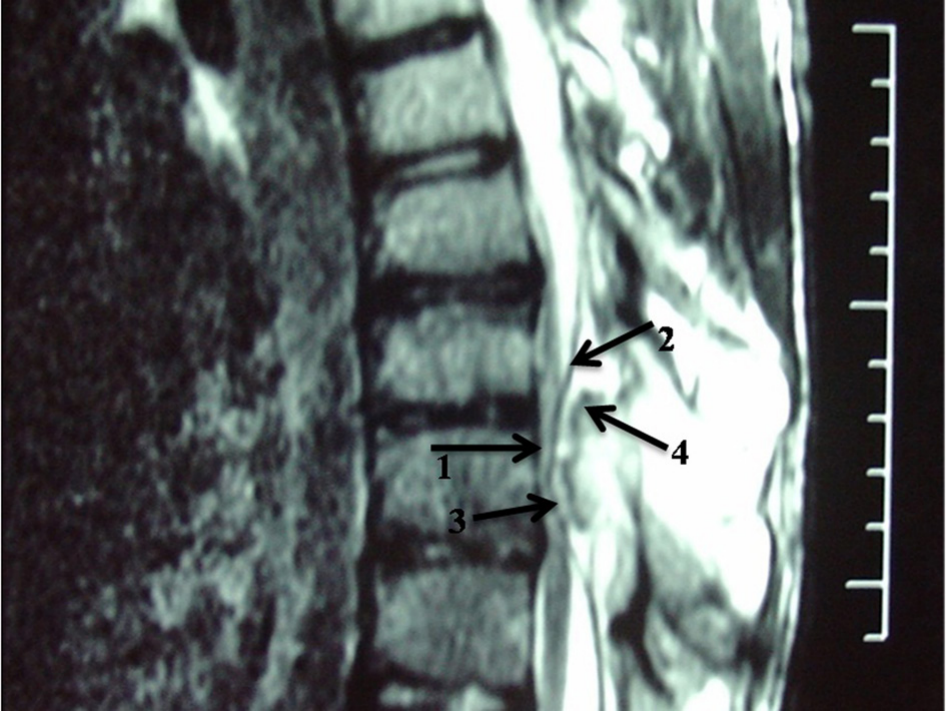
Sagittal T2-weighted MRI. Note the abnormal signal of the thinned and compressed cord (1), the displaced ligamentum flavum (2), the cartilage cap (3) and the cortical bone layer (4)
